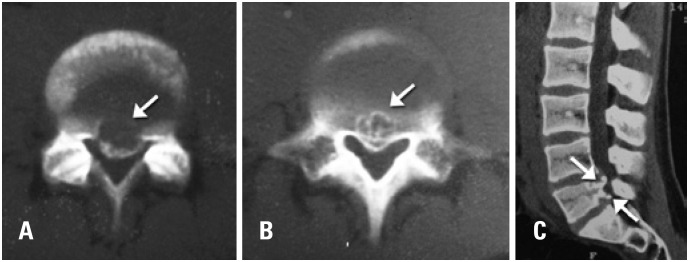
(A and B) Computed tomography and axial slices showing the apophyseal ring fracture (arrow). C) Sagittal slice shows the double injury of apophyseal ring (arrows)
